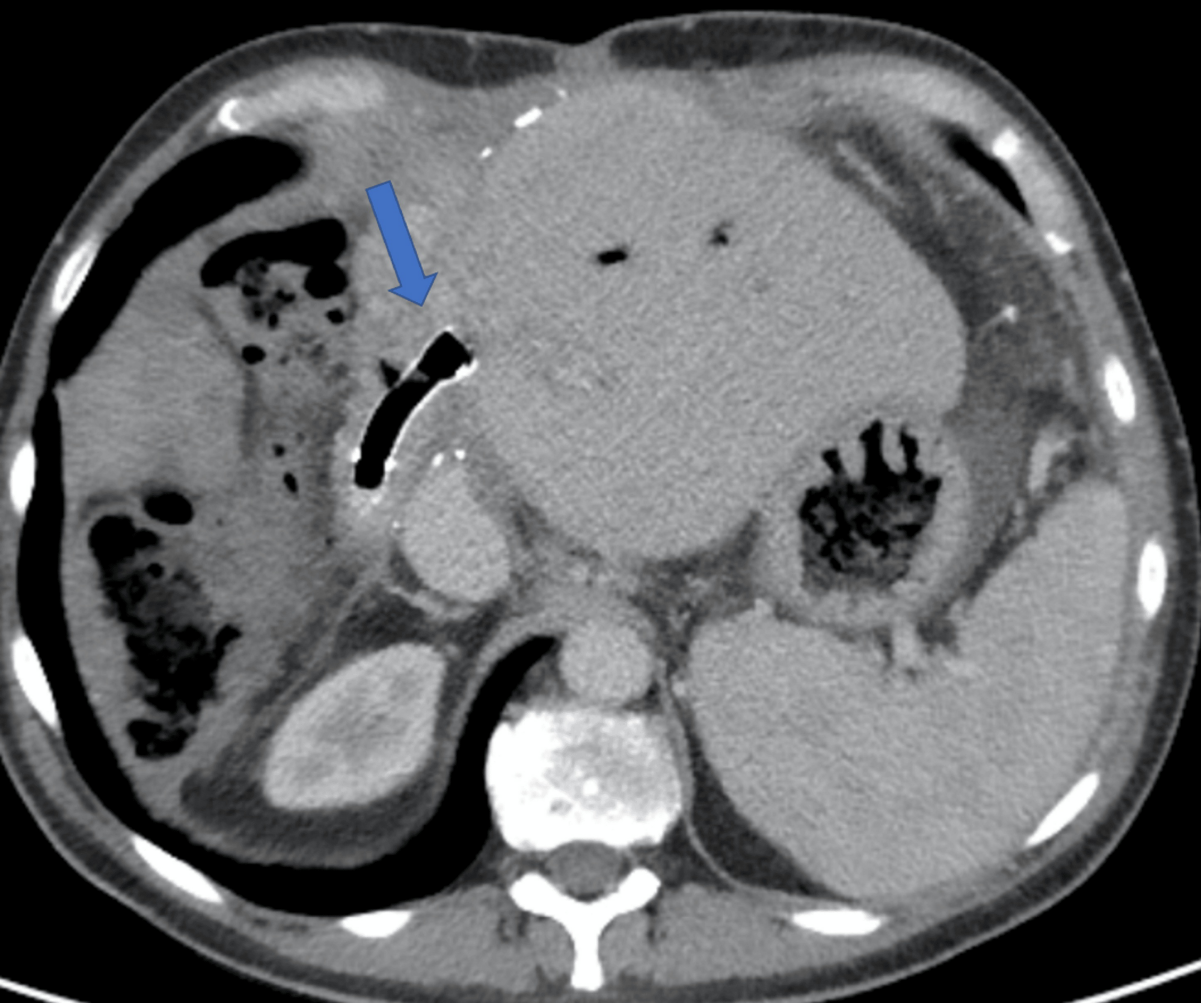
Novel biliary stent insertion via percutaneous trans-jejunal approach The satisfactory position of the metal stent (arrow) with CT demonstrates aerobilia and restored bilio-enteric drainage. CT: computed tomography
